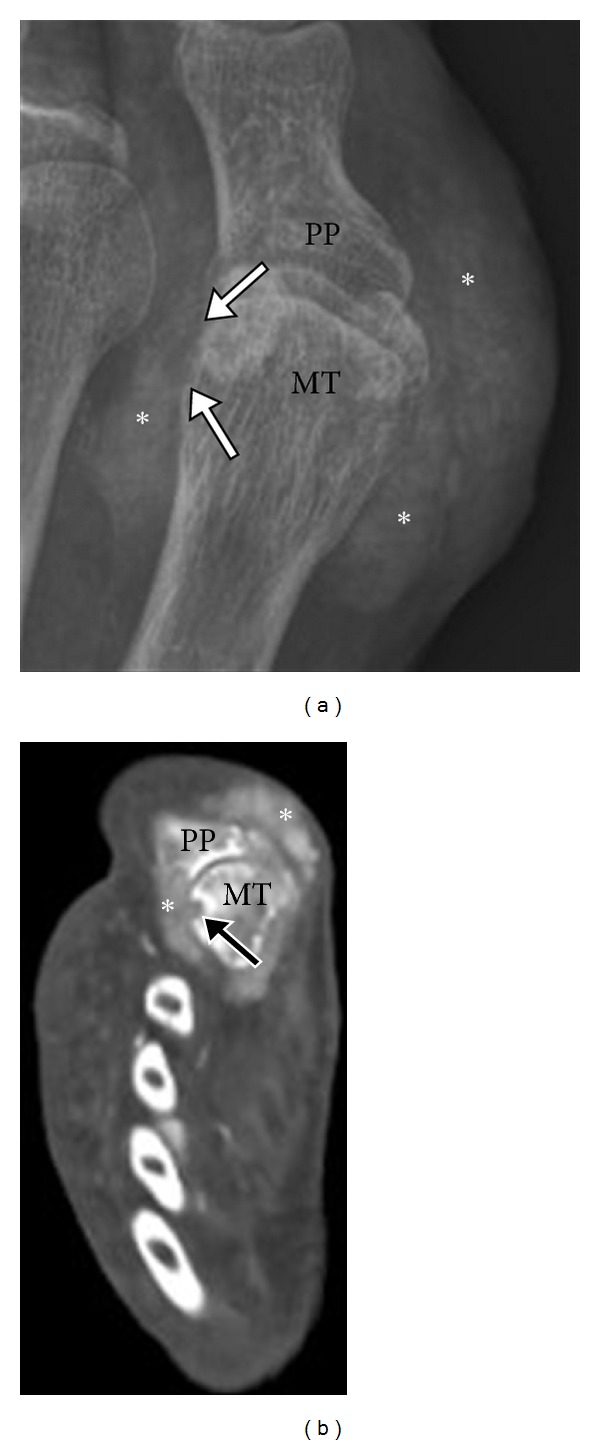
Tophaceous gout on CT. Anteroposterior radiograph (a) of the 1st MTP joint demonstrates dense soft tissue masses (*) centered on the 1st MTP with erosive changes involving the lateral aspect of the 1st MT head (arrows). Corresponding axial CT image (b) shows periarticular high attenuation soft tissue deposit adjacent to the first MTP joint (*) with focal cortical erosion (arrow). MT: metatarsal head; PP: proximal phalanx.
